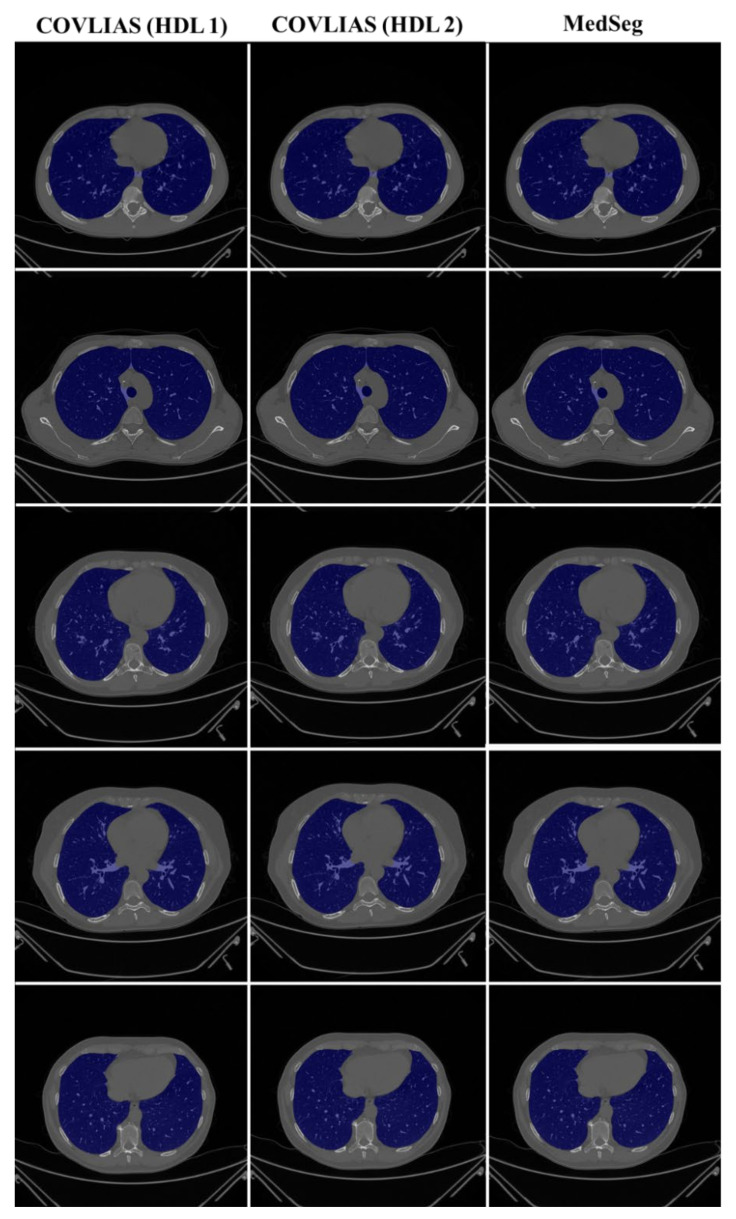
COVLIAS vs. MedSeg: Segmented mask (blue) on the Control CT lung image (large lung).
